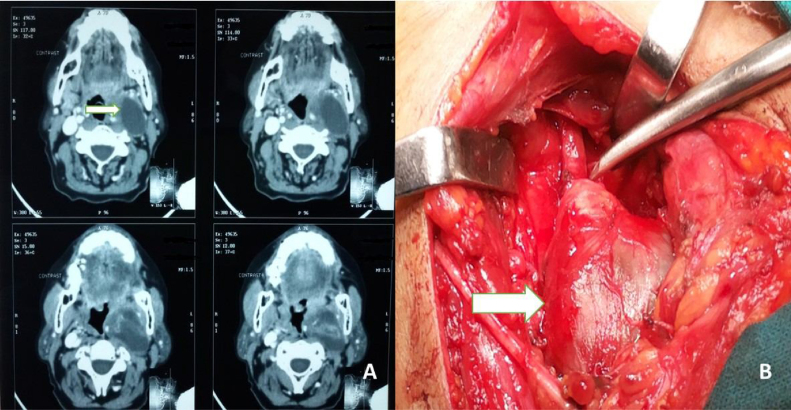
(A) Image showing the axial section of Contrast CT Scan of Vagal Schwannoma. The white arrow pointing towards the right side shows the well-encapsulated schwannoma with cystic change and central enhancing solid component. Vagal schwannoma separates the carotid anteromedially and internal jugular vein anterolaterally. (B) An intraoperative picture of vagal schwannoma is shown by the white arrow pointing towards the right side.
